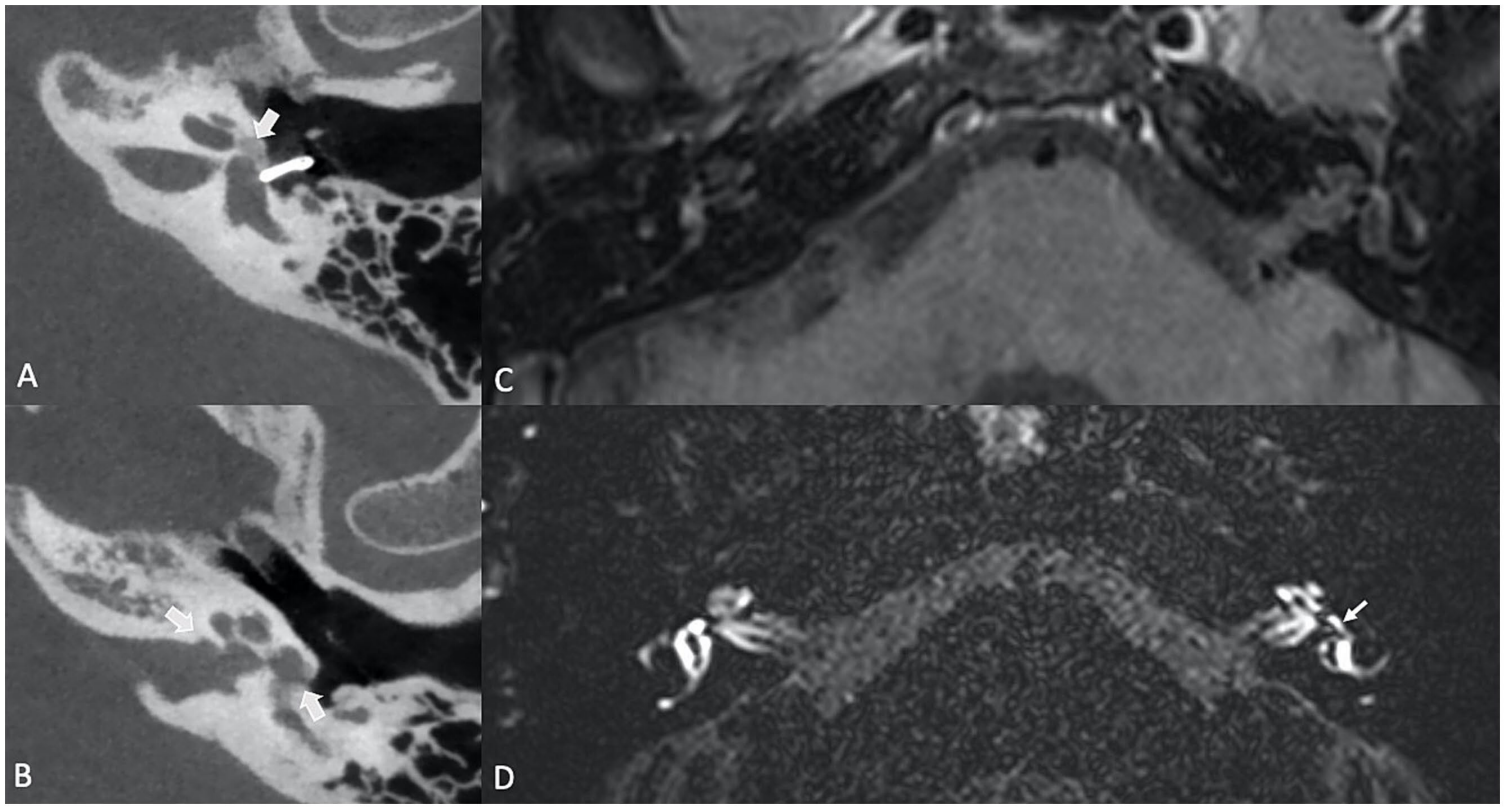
(A,B) Axial high resolution temporal bone Cone Beam CT (CBCT) in a patient with longstanding fenestral and retrofenestral otosclerosis, presenting with otospongiotic plaques at the fissula ante fenestram, the cochlea and the internal auditory canal (thick arrows) and a piston. (C) Contrast-enhanced, axial T1-weighted MR sequence and (D) Contrast-enhanced, delayed SPACE FLAIR MR sequence, showing a cochlear hydrops (grade 1) with irregular dilatation of the scala media, partial obstruction of the scala vestibuli and a vestibular hydrops grade 2 (small arrow) with a beginning of confluence of the enlarged saccule and utricle.
